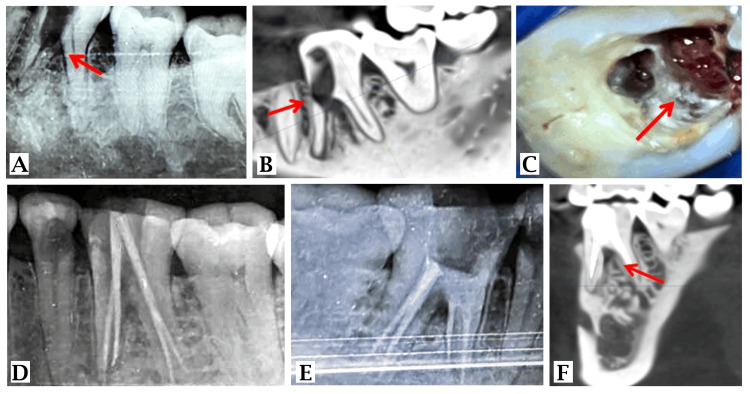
Radiographs and clinical photographs taken preoperatively, intraoperatively, and postoperatively 1A: intra-oral periapical radiograph of 36; 1B: CBCT with 36; 1C: granulation tissue at the perforation site; 1D: mastercone radiograph with 36; 1E: immediate post-operative X-ray after obturation and biodentine placement; 1F: six-month follow-up CBCT CBCT: cone-beam computed tomography
